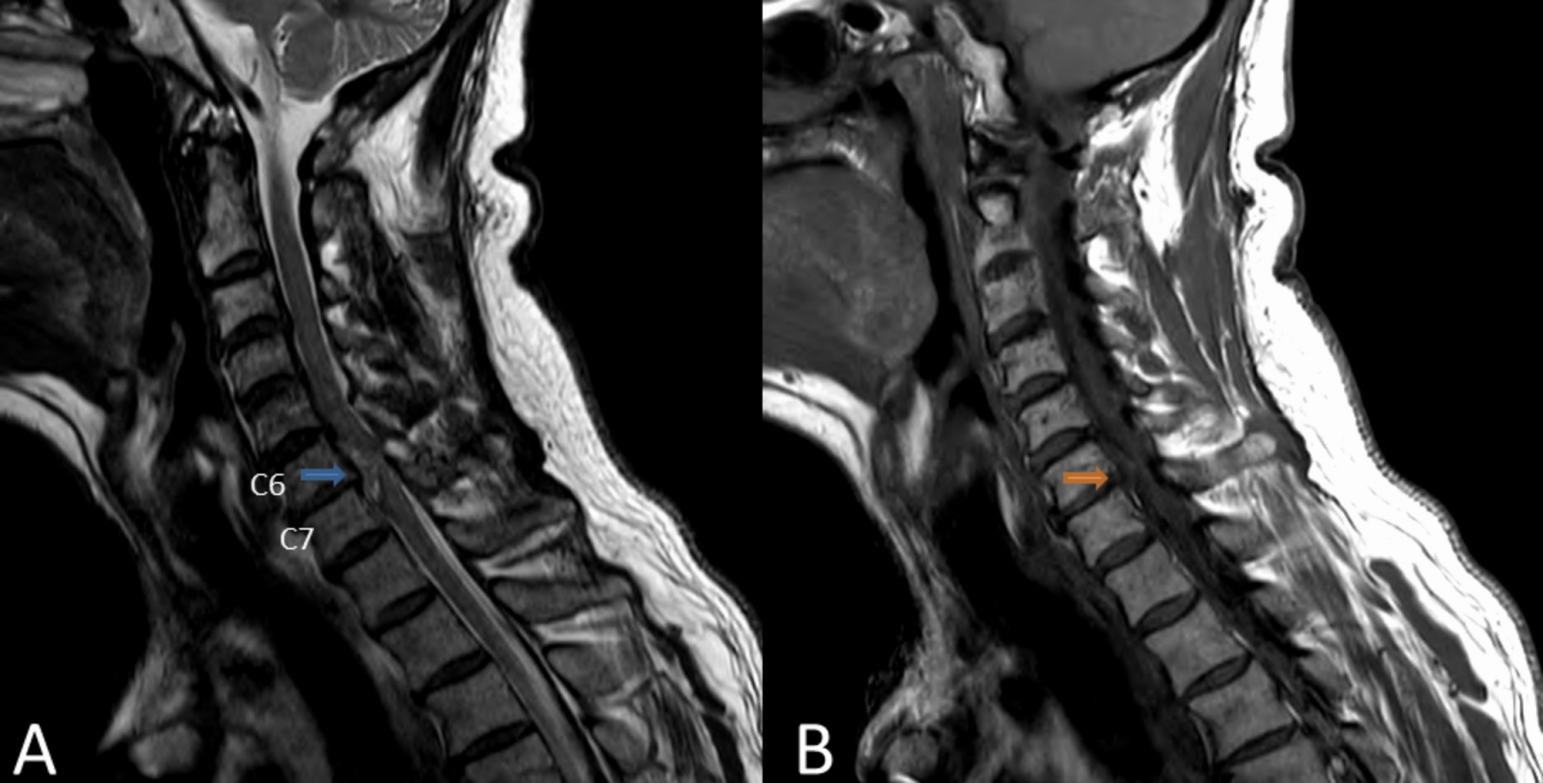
Sagittal MRI Sagittal T2 (A) and T1 (B) MRI demonstrated a cervical disc herniation at C6-C7 (arrows). The lesion was also read as a possible meningioma. The lesion extends behind each of the vertebral bodies rostrally and caudally. The spinal cord is severely compressed without evidence of myelomalacia.
